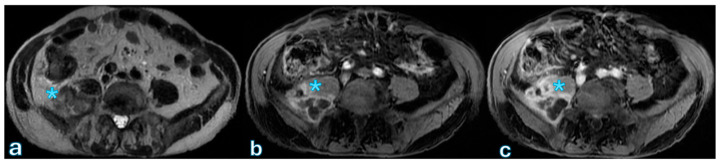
The MR T2-weighted image sequences (a), T1-weighted sequence after administration of contrast-enhanced arterial phase (b), and late phase (c) reveal a psoas muscle lesion with peripheral enhancement, which is consistent with an abscess indicated with blue asterisk. This finding was observed in a patient with fever and abdominal pain, 1 month after surgery for pancreatic adenocarcinoma localized to the body.
